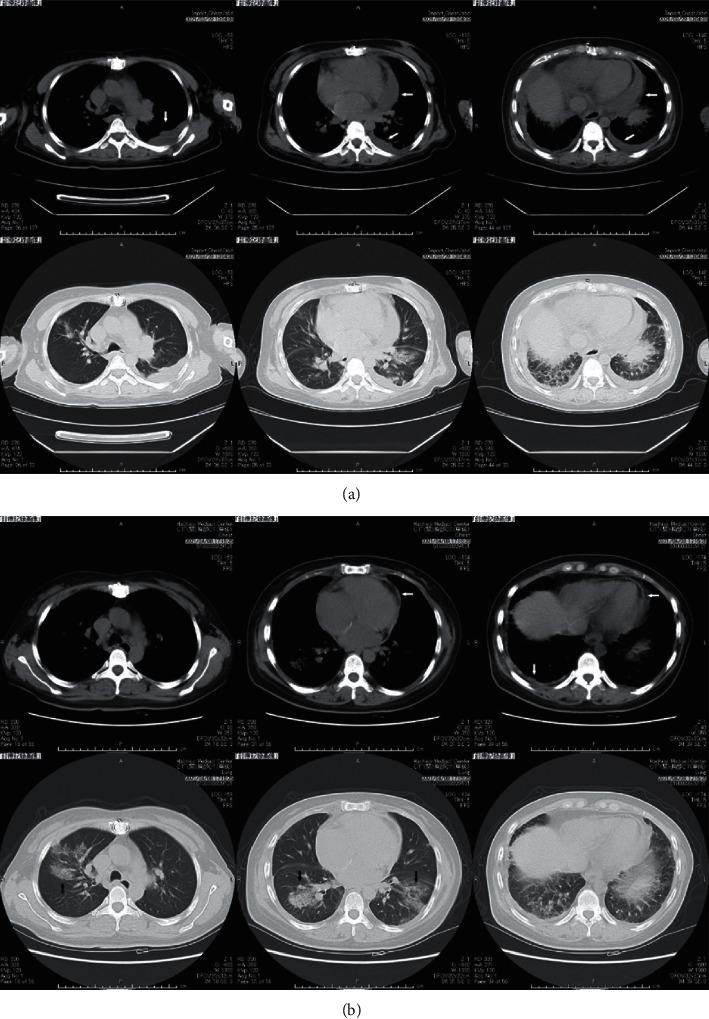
Chest plain computed tomography scan image performed at the referral hospital (a) at the time of admission to our hospital (b). The white arrows indicate the accumulated pleural effusion and pericardial fluid, or thickened pleura and pericardium. The black arrows indicate exacerbated ground-glass opacities.
